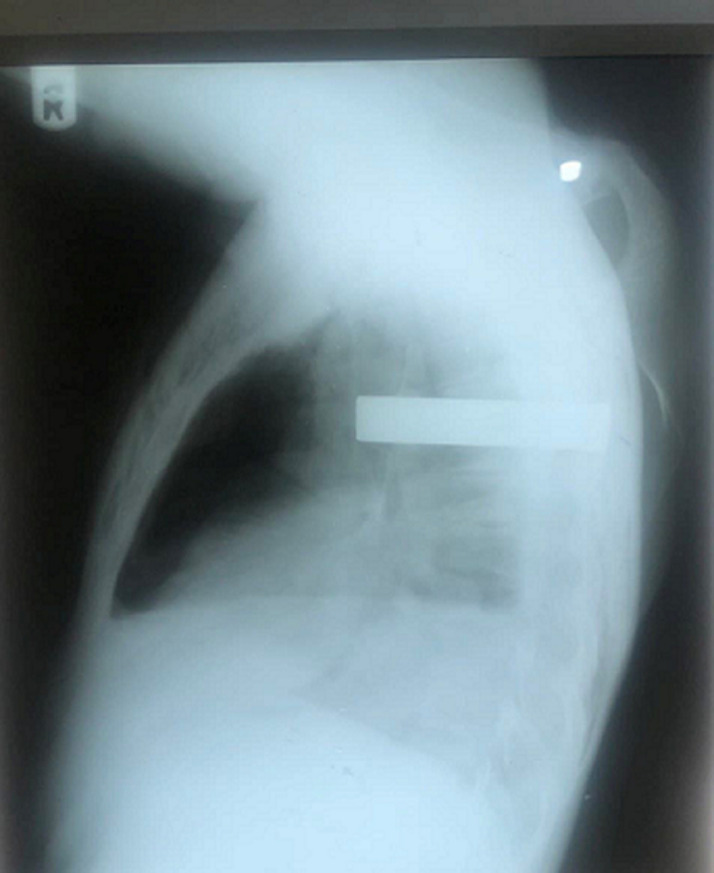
lateral chest radiograph of the second case
